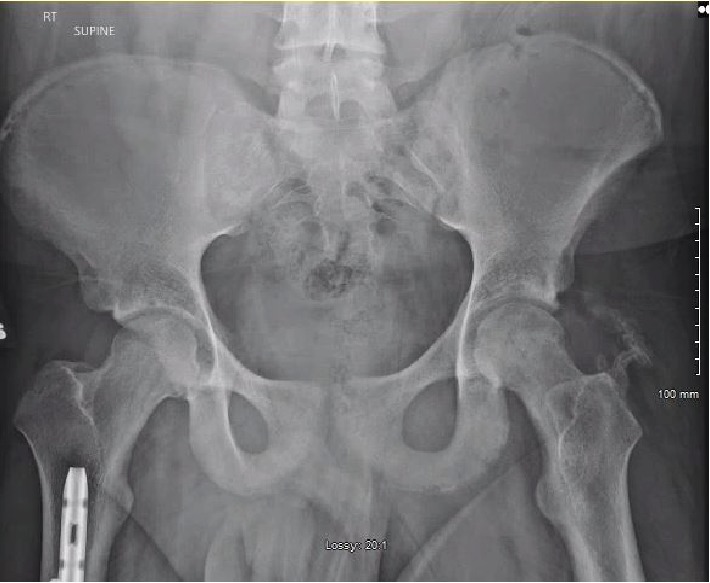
Follow-up AP pelvis radiograph at 9 weeks postinjury demonstrating concentric reduction of the left femoral head with heterotopic ossification without evidence of femoral head collapse.
